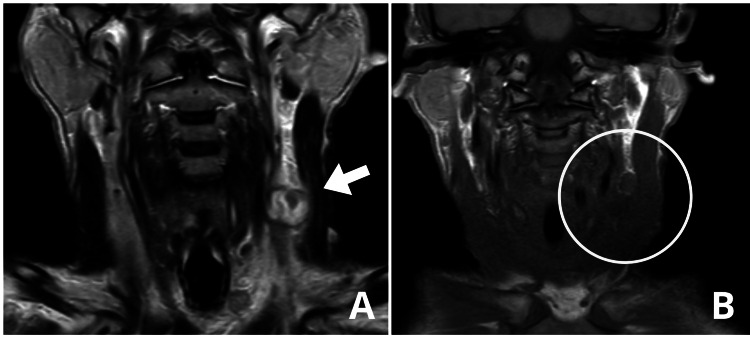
MRI of the head MRI with contrast in T1-weighted sequence (A) shows an oval-shaped image with well-defined borders (white arrow), located in the region of the left internal jugular vein, consistent with thrombophlebitis. MRI with contrast in T2-weighted sequence (B) displays a heterogeneous image predominantly hyperintense with a hypointense peripheral halo, measuring approximately 2.4×2.1 cm (white circle).
